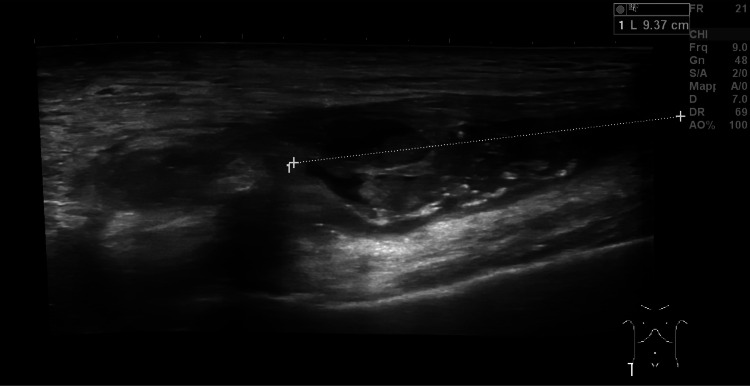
Preoperative ultrasound imaging of the periprosthetic fluid collection
